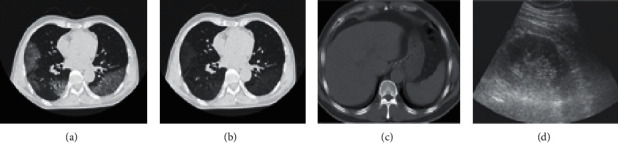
CT and ultrasound images. (a) The chest CT image of COVID-19 cases. (b) The segmented image of the pneumonia-infected area. (c) CT of the abdomen. (d) Ultrasound of the abdomen.
